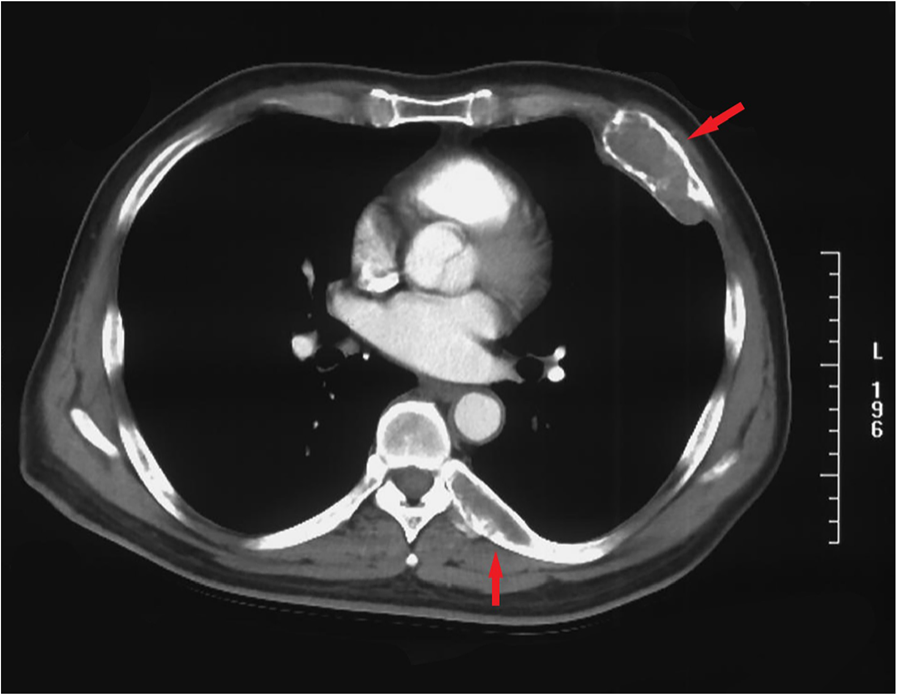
Axial computed tomography image shows the expansive lesion with cortical destruction that originates from the left anterolateral fifth rib, and reveals a well-defined radiolucent unilocular mass that expands the cortical bone of the left posterior eighth rib
